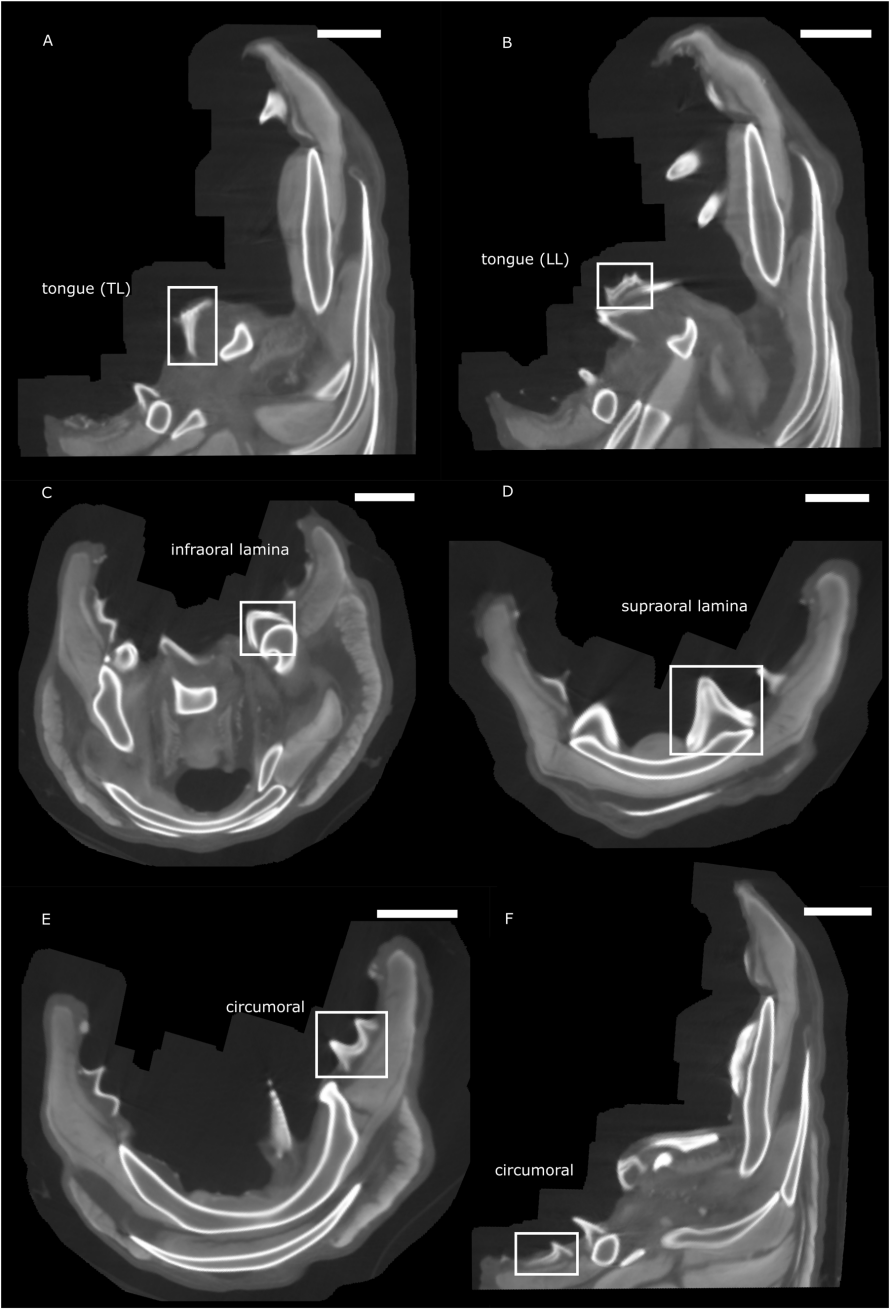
micro‐CT tomography data of *M. mordax* adult toothlets. (A, B) Sections through the tongue teeth*:* (A) Transverse lamina (TL) with one replacement cone. (B) Longitudinal lingual lamina (LL) with one replacement cone. (C) Section through the infraoral lamina with one replacement cone. (D) Section through a three‐cuspid tooth of the supraoral lamina with one replacement cone. (E) Section through a two‐cuspid circumoral tooth in the lateral field with one replacement cone. (F) Section through a single‐cuspid circumoral tooth in the posterior field with one replacement cone. Rectangles mark the respective tooth. Scale bars represent: 3000 μm (A–C, E), 1000 μm (D), 2000 μm (F).
